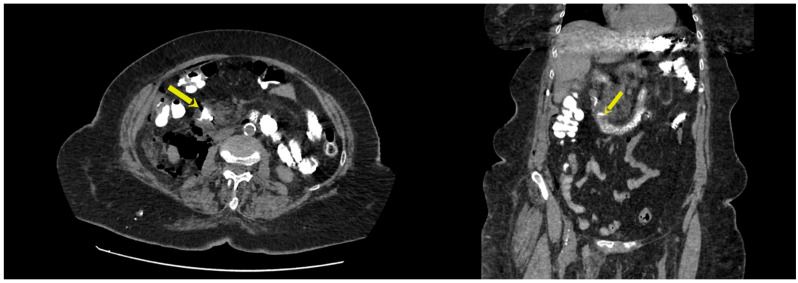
The results of a CT scan with oral contrast in a patient with a Stapfer II perforation treated intraprocedurally with plastic stent placement and endoclips. Yellow arrows point to the papillary region depicting lack of contrast extravasation.
